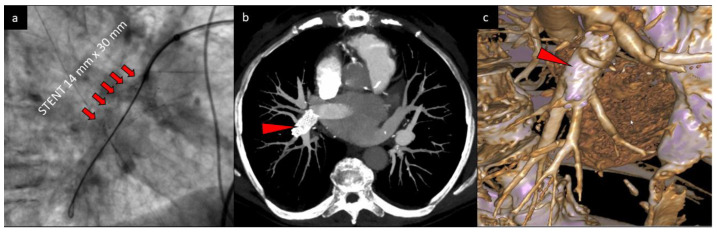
The final result of BPA and stent implantation (marked by red arrows) presented in fluoroscopy (a), computed tomography (b), and 3D reconstruction (c).
